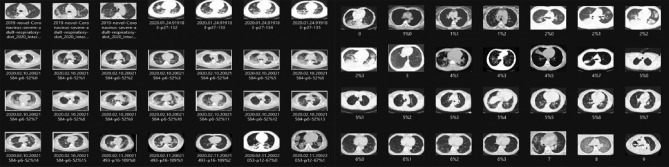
349 positive COVID-19 diagnosis CT and 397 negative medical imagery with 97% accuracy.
